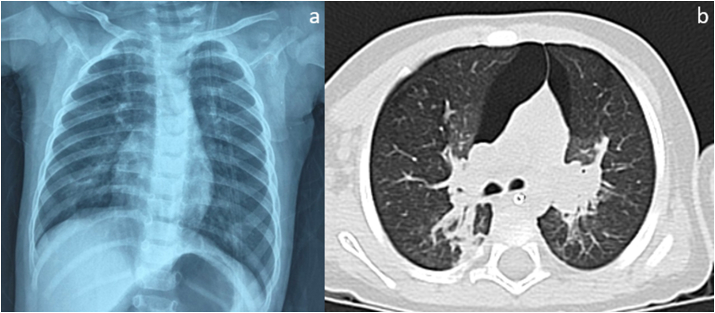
a. CXR showing no abnormalities. b. Axial sequence of a lung window chest-CT revealing a bilateral large pneumothorax with an interstitial alveolar pneumonitis.
